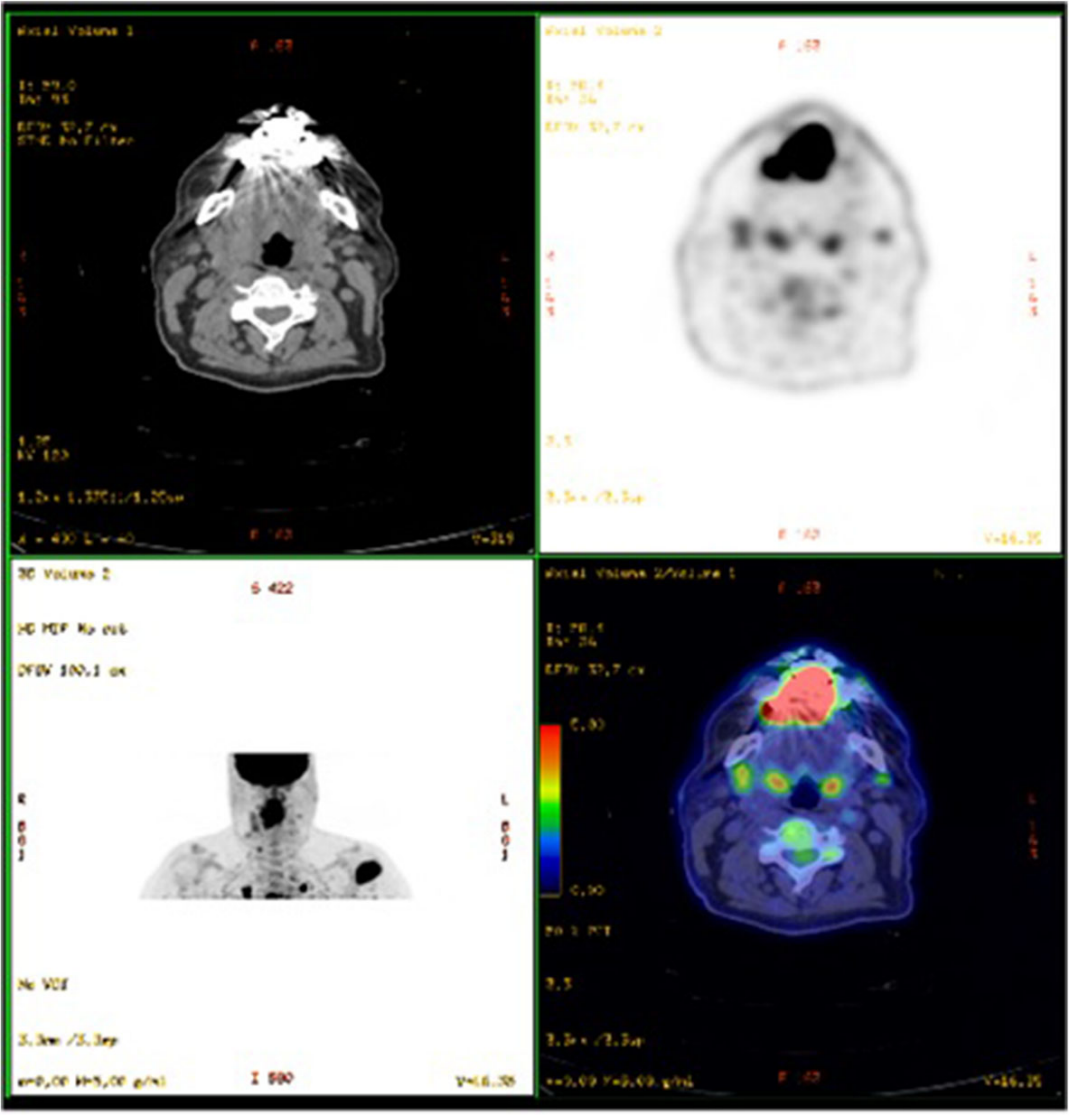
Positron emission tomographic-computed tomography scan shows a tongue lesion
